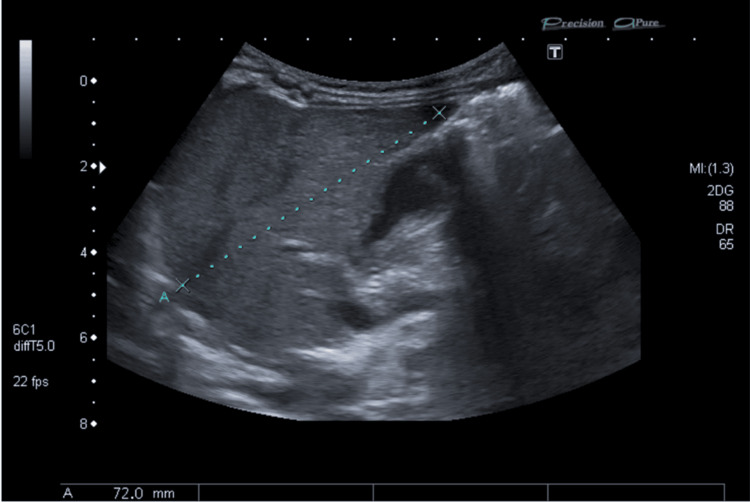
Abdominal ultrasound Abdominal ultrasound shows the liver of normal size, with regular contours, homogeneous echostructure, and normal reflectivity. It measures approximately 72 mm in the longitudinal axis in the right lobe. There is no dilation of the intra- and extrahepatic bile ducts. No suggestive signs of biliary atresia (such as the absence of a normal gallbladder and presence of triangular cord sign) or anatomical alterations were present. The absence of biliary dilation ruled out obstructive hyperbilirubinemia.
